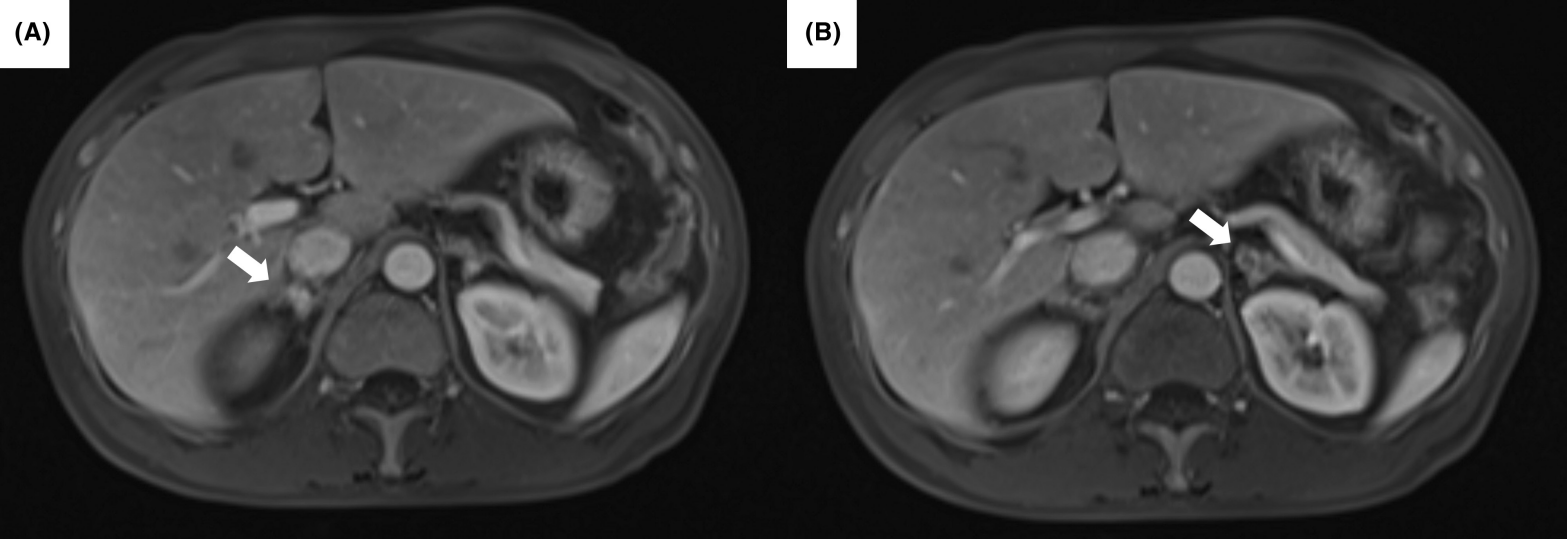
Abdominal magnetic resonance imaging with contrast enhancement. Magnetic resonance imaging showed that the adrenal glands were enlarged but smaller than on computed tomography on admission (A) and (B). Although post‐contrast T1‐weighted images revealed an area of partial low density, the contrast enhancement of the bilateral adrenal glands was good.
